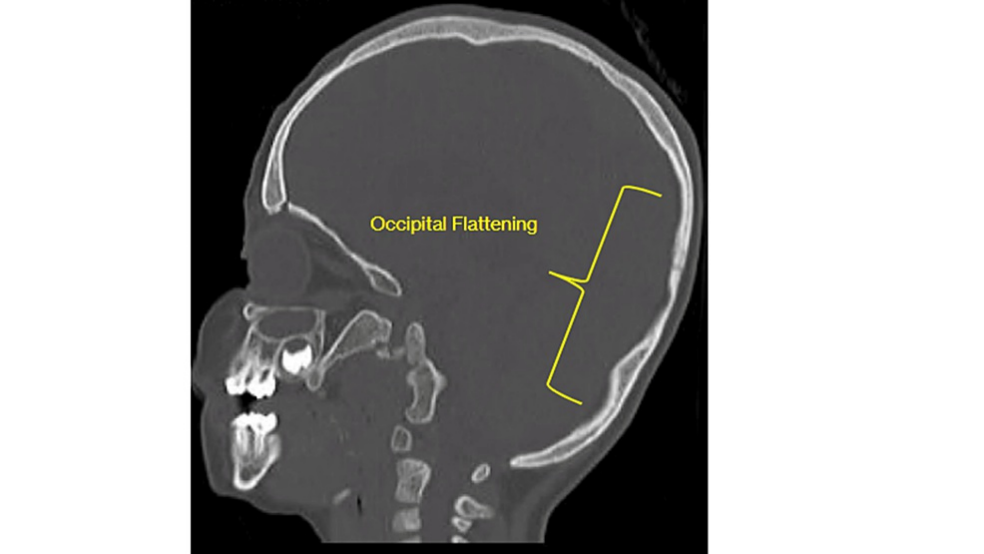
Head CT sagittal view on bone windows demonstrating occipital flattening.
